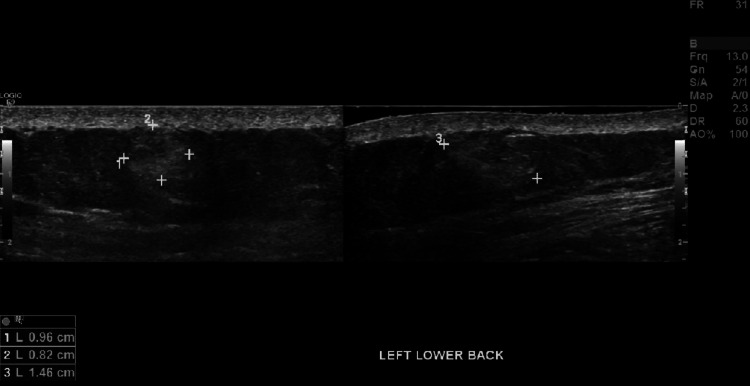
Ultrasound image of a 1.46 x 0.96 x 0.82 cm left lower back soft tissue mass
